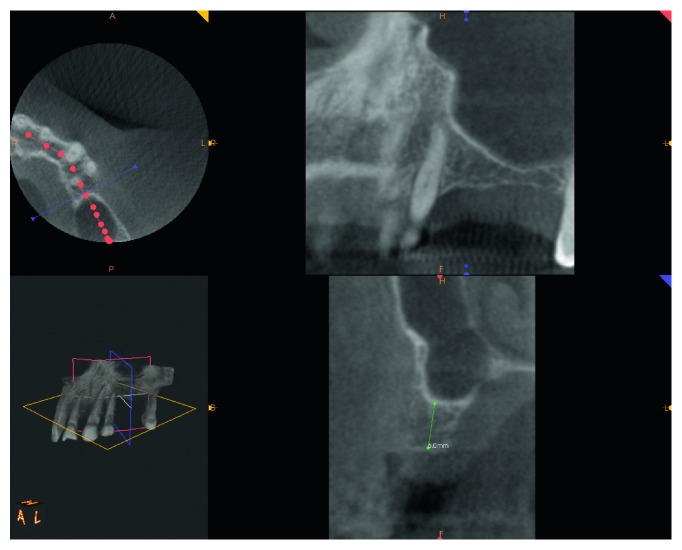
CT scan pre-op showing 6 mm Bbh at mesial site.
